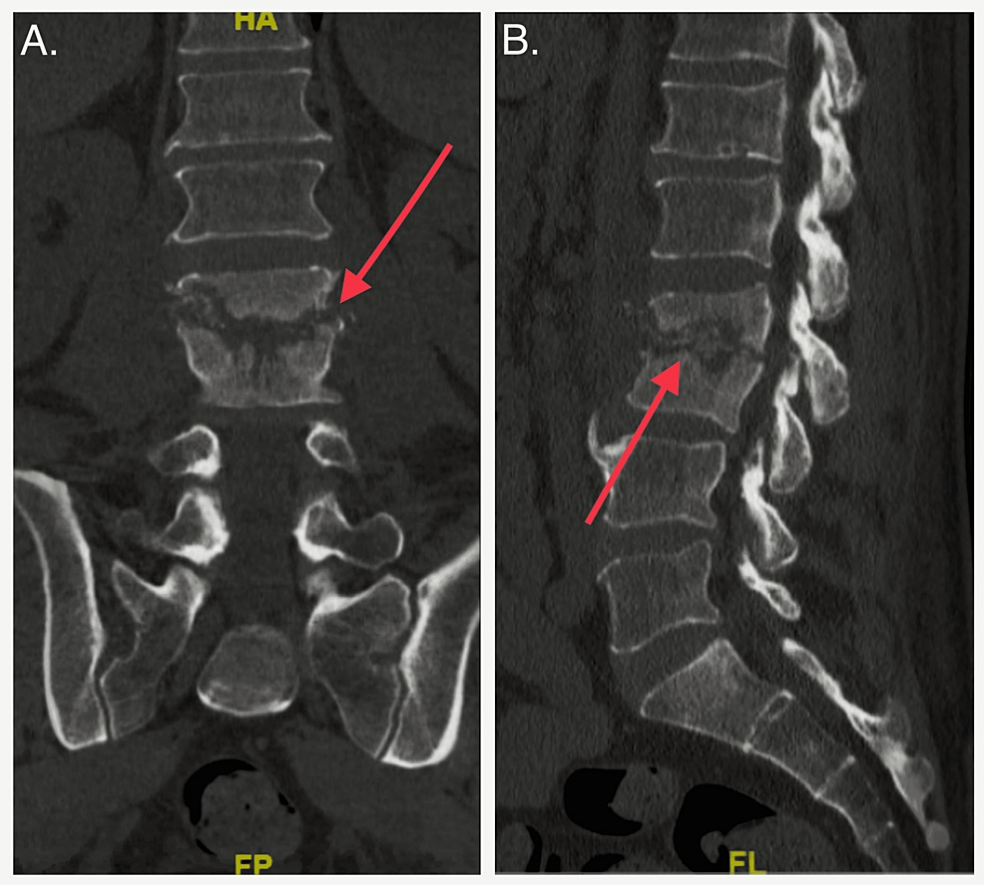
Multi-planar axial images from CT showing cortical destruction of the inferior endplate of L2 and superior endplate of L3 with a loss of intervertebral disc space, consistent with discitis with osteomyelitis. There are bony fragments with retropulsion into the spinal canal at L2/L3 with moderate to severe spinal canal stenosis (red arrows). CT: computed tomography.
